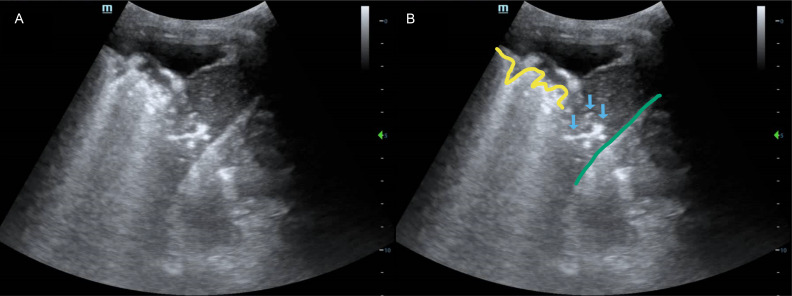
A) Unlabeled point of care ultrasound (POCUS) image of Supplementary Material S1 at 0:00. B) The structures of interest are highlighted. The curved green line highlights what was falsely presumed to be the diaphragm. The blue arrows show hyperechoic foci against a soft tissue density, initially interpreted as “air bronchograms.” The yellow line highlights the irregular hyperechoic line with distal reverberation artifact, initially interpreted as “shred sign.”
